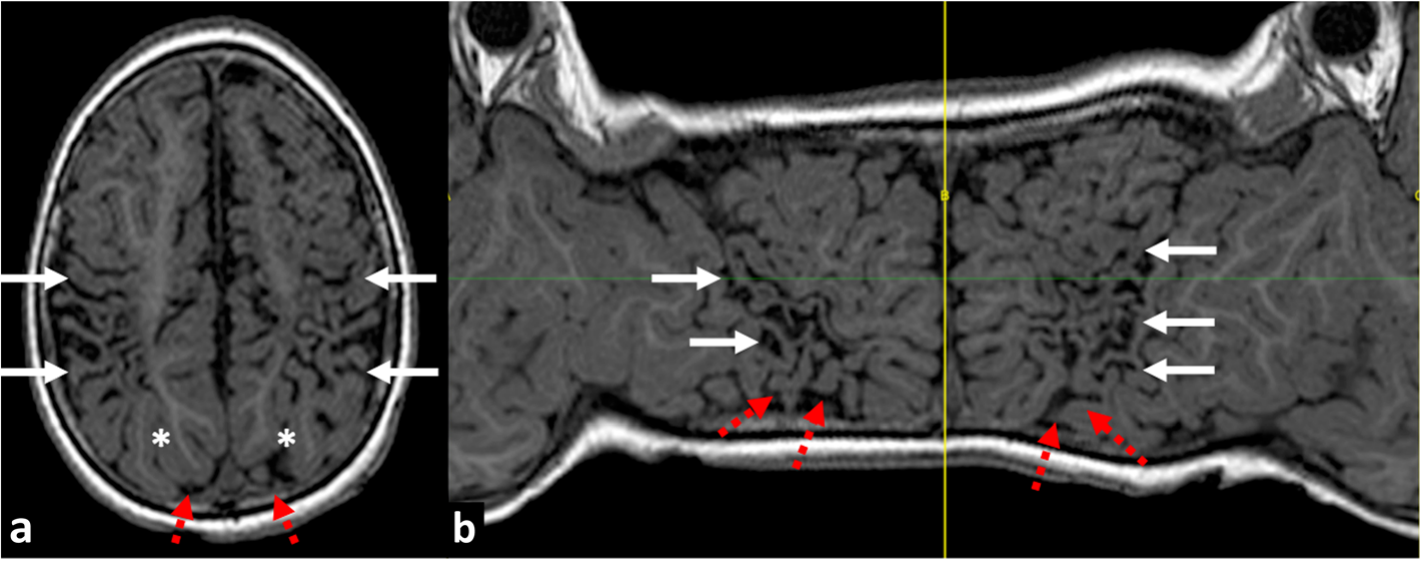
a Axial reference T1W image and (b) flat earth Mercator map derived from curved reconstructions of 3D T1-weighted images in a 6-year-old girl demonstrating atrophy and ulegyria in the peri-Sylvian (white arrows) and posterior watershed (red dashed arrows) distribution in a child who suffered partial-prolonged hypoxic-ischaemic injury perinatally at term gestation. Note sparing of the anterior and parasagittal watershed regions as well as the temporal lobe portions of the peri-Sylvian watershed. Also, note the asterisk depicting the apparent discontinuity on the axial image which is clearly shown to be continuous on the Mercator map
